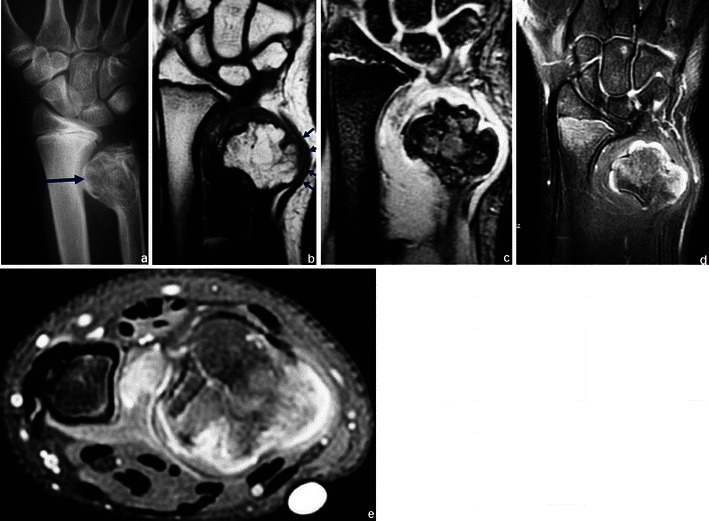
Anteroposterior radiograph (a) and corresponding MRI study (coronal T1 (b), coronal T2FS (c) and coronal (d) and axial post-Gd T1WI (e) of a broad-base osteochondroma of the ulna. T1WI shows normal bone marrow signal intensity inside the lesion, and T2FSWI shows adjacent effusion and oedema of the adjacent soft tissues. The flexor carpi ulnaris tendon is compressed and displaced (arrows in b). The axial post-Gd T1WI (e) shows no compression of the adjacent neurovascular structures
